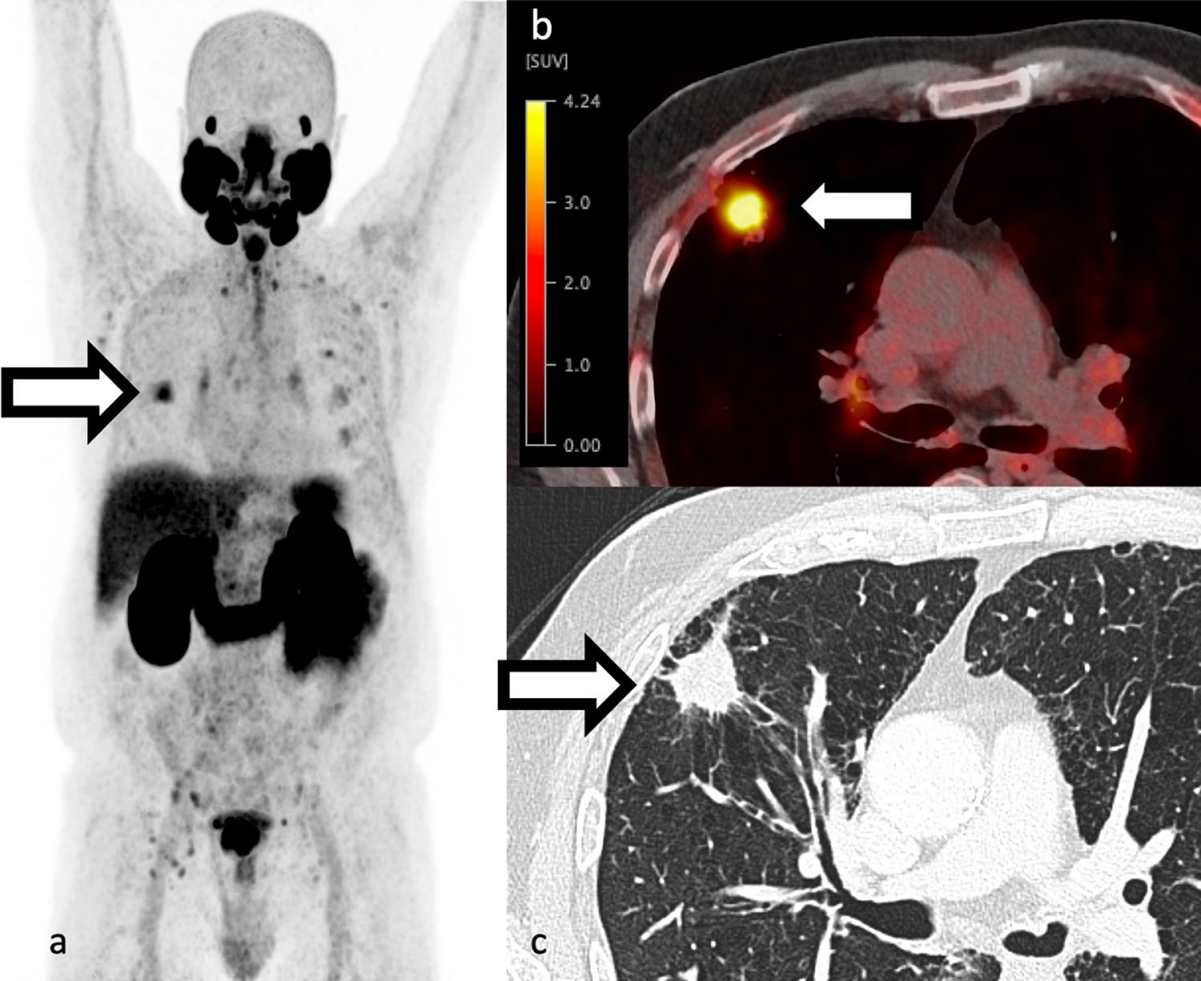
a-c 74-year-old man with a PSA 8.1, Gleason 4+3 prostate cancer. Staging Ga68-PSMA-11 PET findings consistent with T3bN1bMx disease and spiculated 2.6 cm right upper lobe nodule with low radiotracer uptake (SUVmax 5.8) on a background of emphysema (white arrow in panels a–c). Subsequent FNA was consistent with SCC of lung. There is increased uptake within the prostate with known cancer and metastatic pelvic lymph nodes. Several foci of low-level radiotracer uptake without CT correlate in the posterior left sixth rib without a CT or subsequent FDG correlate, favored to be benign. Maximum intensity projection a SUV scale 0–5
